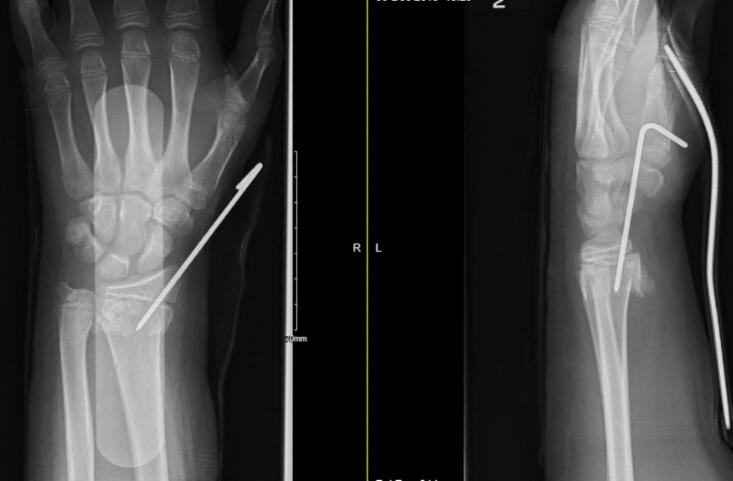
AP and lateral radiograph showing backing out of K-wire.
